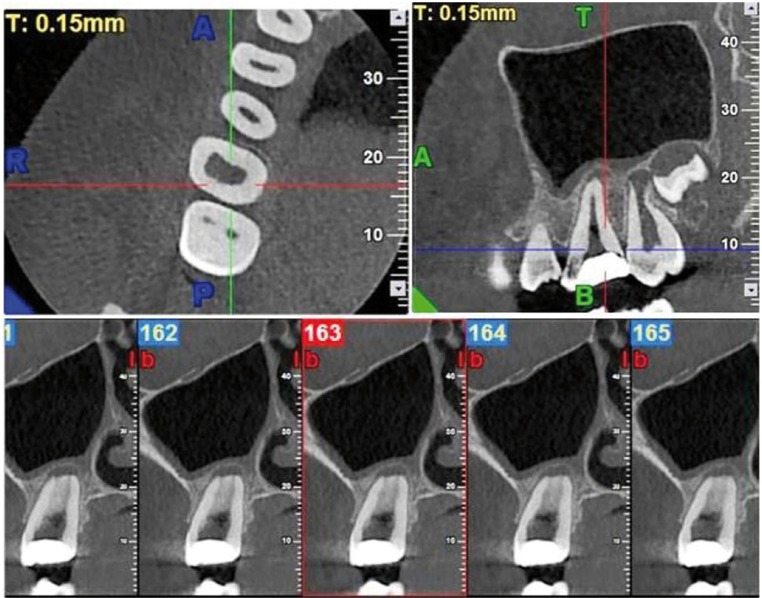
Preoperative occlusal, proximal and buccal CT view of maxillary first molar.
